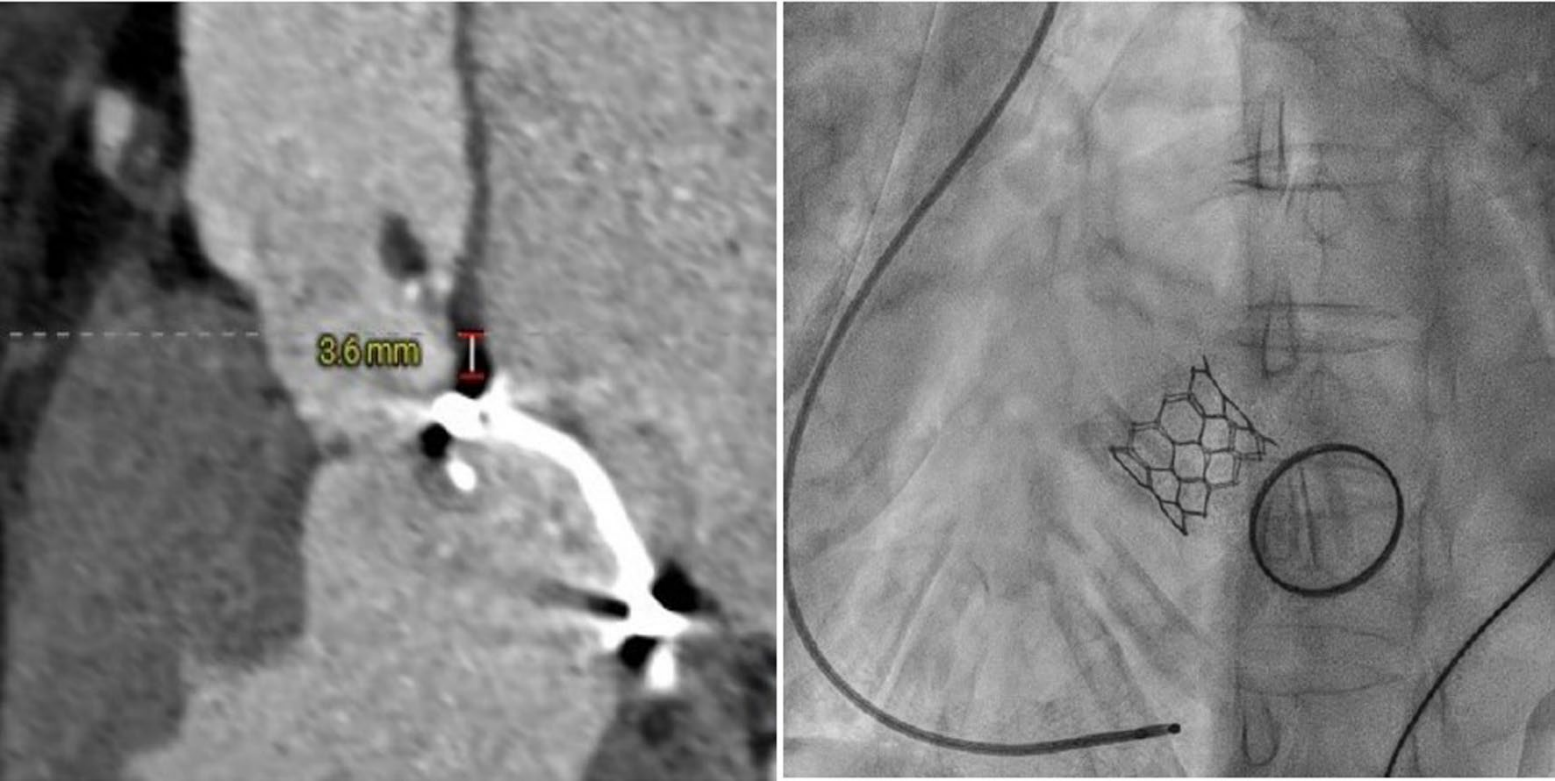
(case 2): On the left, pre-procedure Cardiac CT scan showing the prosthetic mitral valve—aortic annular distance. On the right, post-procedure fluoroscopic image showing deployed balloon expandable THV in the aortic position and a mechanical PHV in the mitral position
